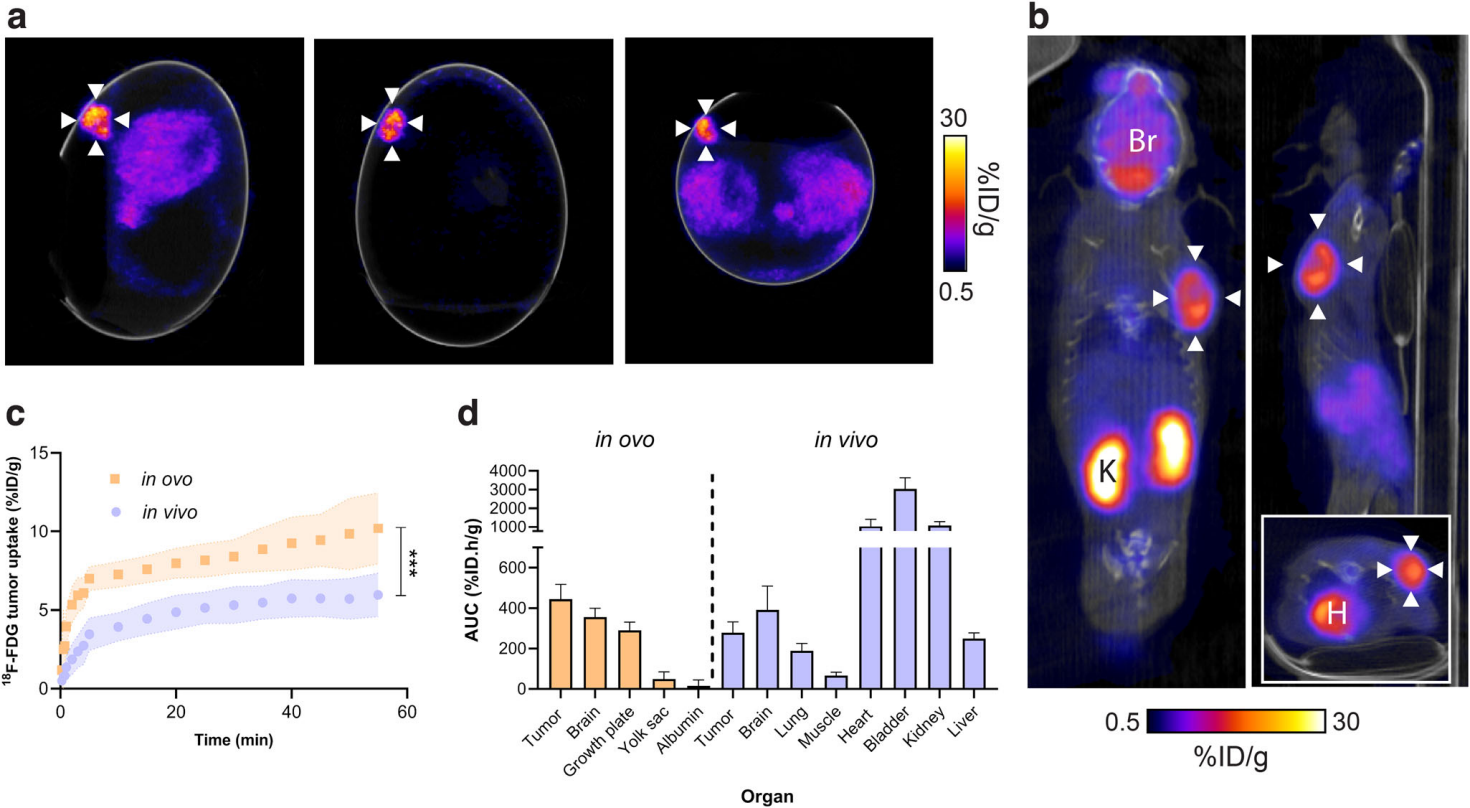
Comparison of in ovo and in vivo ^18^F-FDG
